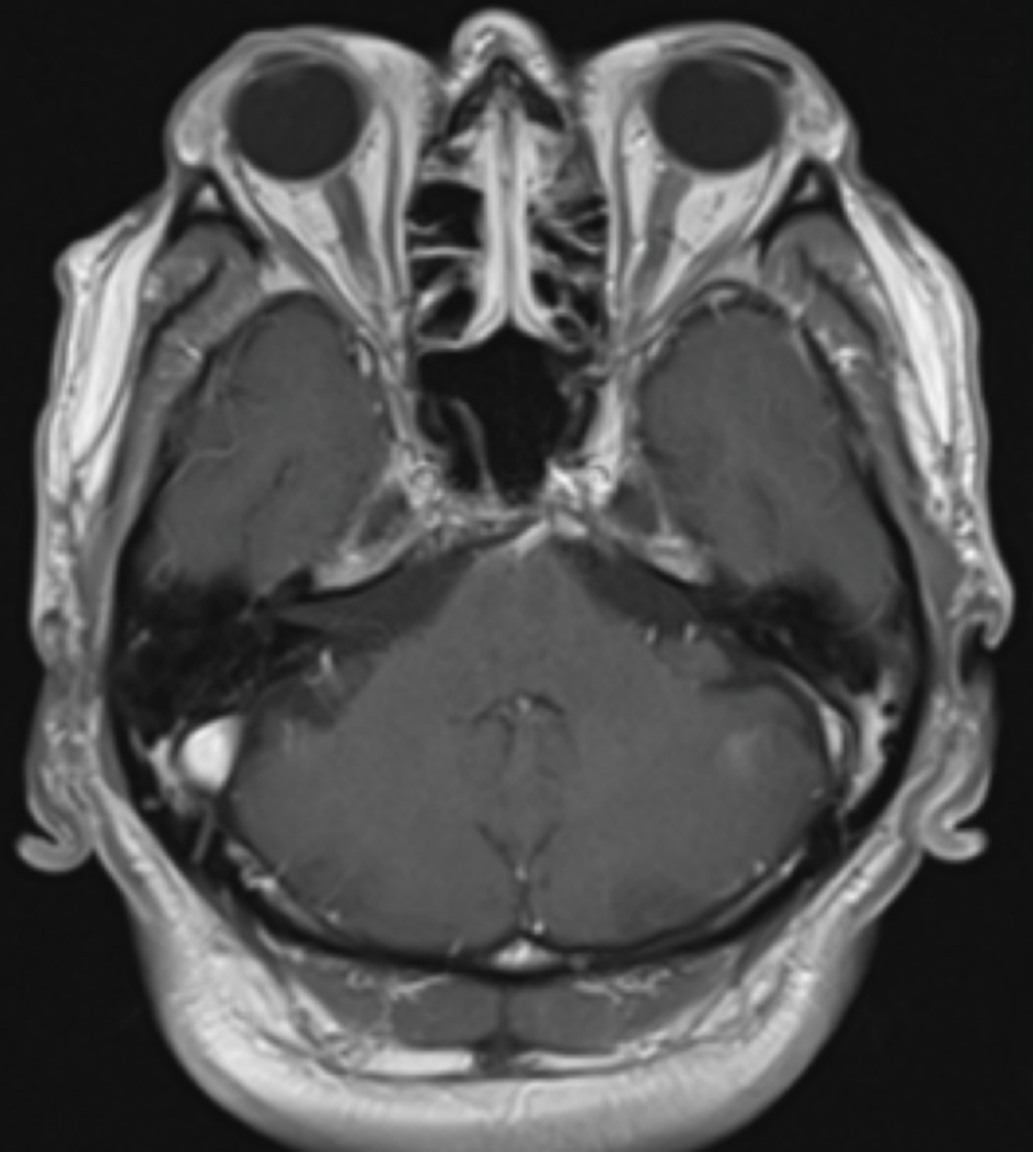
Axial T1 MRI of the brain with contrast from 2014 showing no acute intracranial abnormalities or masses. MRI: Magnetic resonance imaging
